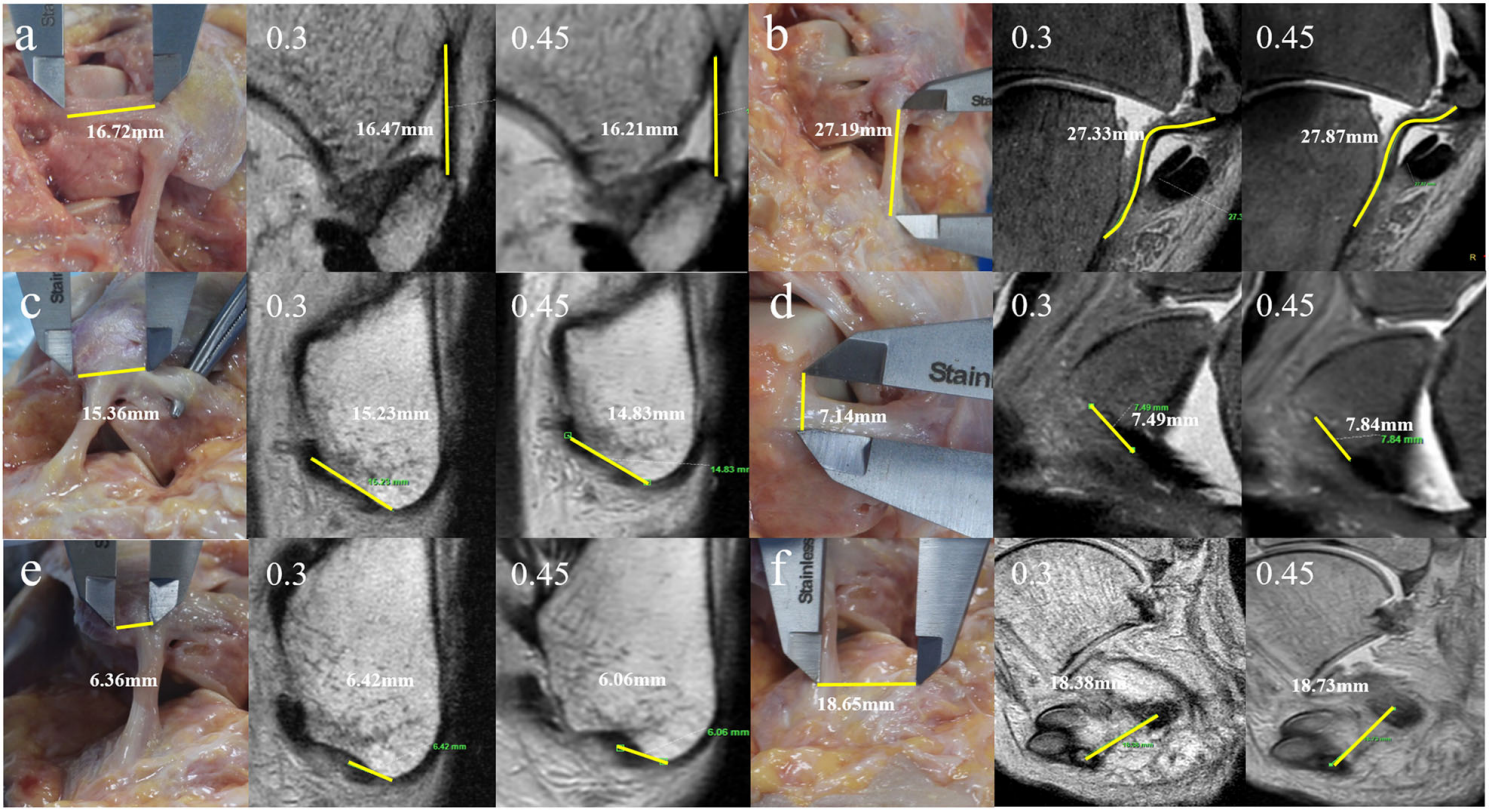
Measurements from the autopsy data and MRI in spatial resolutions of 0.3 × 0.3 × 0.3 mm^3^ and 0.45 × 0.45 × 0.45 mm^3^. a The entire length of anterior talofibular ligament (ATFL). b The entire length of calcaneofibular ligament (CFL). c From the fibular origin of the ATFL to the tip of the fibula. d From the talar insertion of the ATFL to the bare zone of the talus. e From the fibular origin of the CFL to the tip of the fibula. f From the calcaneal insertion of the CFL to the peroneal tubercle
